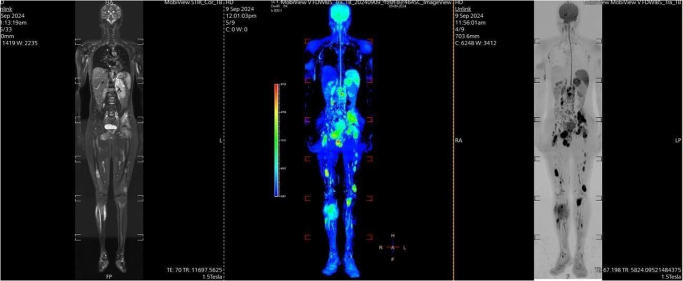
A whole-body diffusion weighted MRI shows multiple lesions in the spine, pelvic bones and lower limb skeleton.
